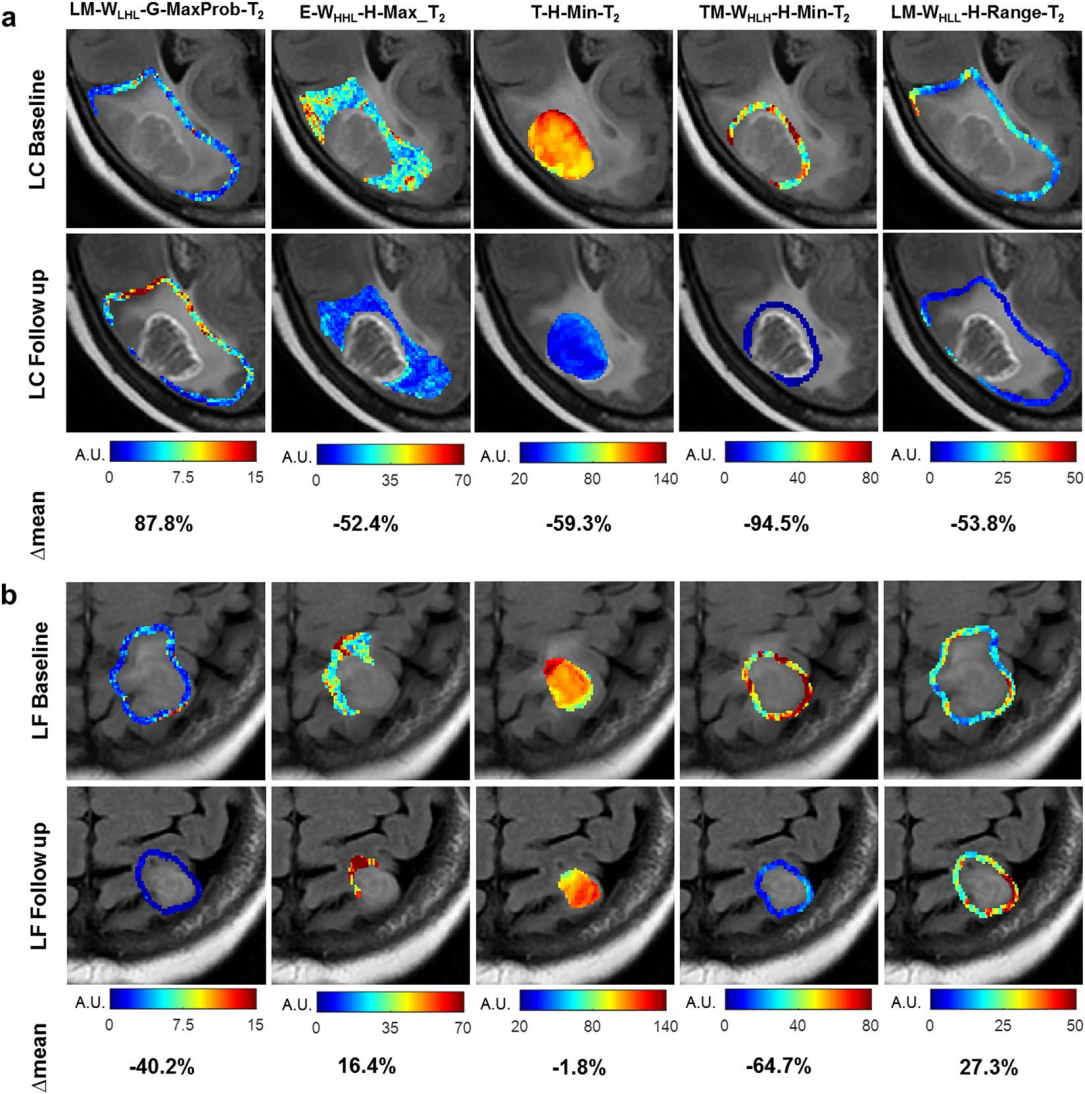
Representative parametric maps of the features in the optimal qMRI biomarker for the overall LC/LF outcome. The parametric maps show the spatial variations in the features derived from the MR images acquired at the baseline and the first follow up for representative tumours with LC (a) and LF (b) outcomes. The mean relative change from the baseline at the first follow up (∆mean) is given for each feature. Biomarker abbreviations: LM-W_LHL_-G-MaxProb-T2: Lesion-Margin_Wavelet_I_LHL__GLCM_MaximumProbability_T2; E-W_HHL_-H-Max_T2: Edema_Wavelet_I_HHL__Histogram_Maximum_T2; T-H-Min-T2: Tumour_Histogram_Minimum_T2; TM-W_HLH_-H-Min-T2: Tumour-Margin _Wavelet_I_HLH__Histogram_Minimum_T2; LM-W_HLL_-H-Range-T2: Lesion-Margin _Wavelet_I_HLL__Histogram_Range_T2.
